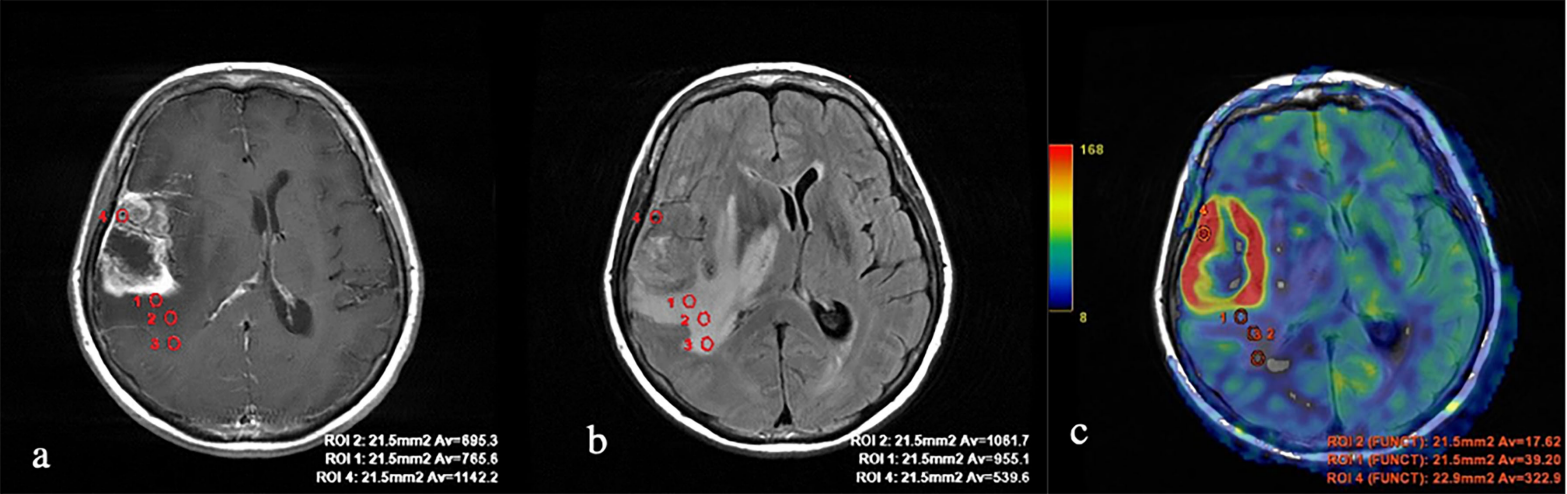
Glioblastoma. (A) T1 with contrast enhancement; (B) T2-FLAIR; and (C) TBF blood flow maps aligned with T2-FLAIR. Measurements of hemodynamic parameters of tumor blood flow in the peritumoral zone are shown.
